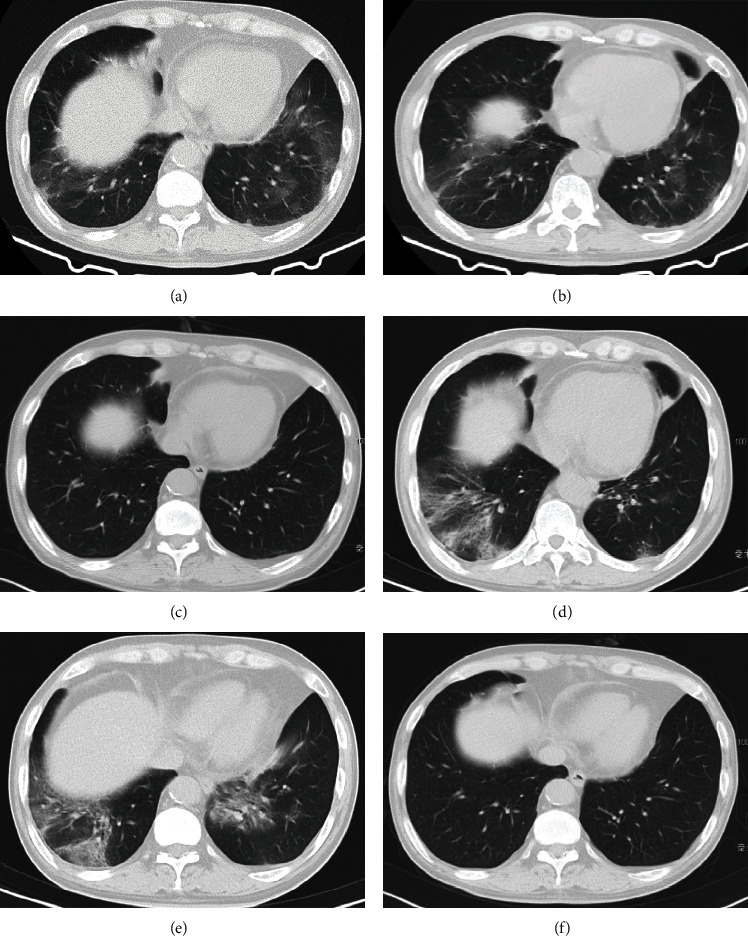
Chest CT scans of the patient with recurrent SARS-CoV-2 pneumonia. (a) First episode of SARS-CoV-2 pneumonia. (b) Second episode of SARS-CoV-2 pneumonia, and (c) improvement after treatment. (d, e) Third episode of SARS-CoV-2 pneumonia, and (f) improvement after treatment with Paxlovid and tix-cil.
